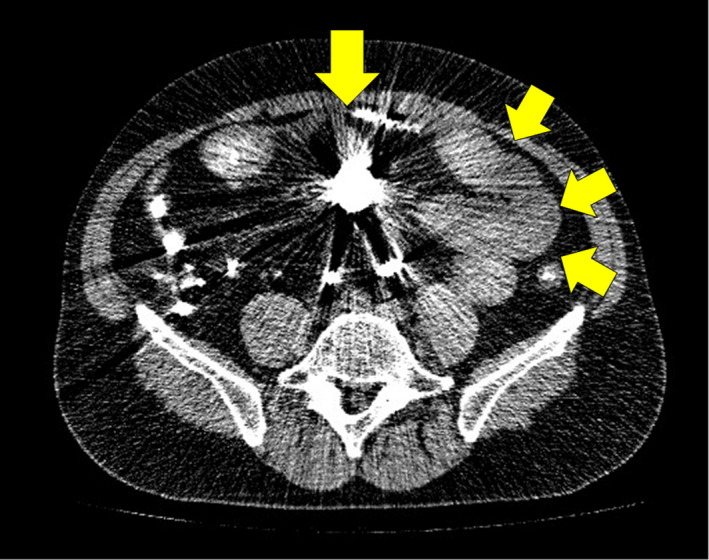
Abdominal CT with contrast material showing distended small bowel loops, barium accumulation (arrow), and small ascites
